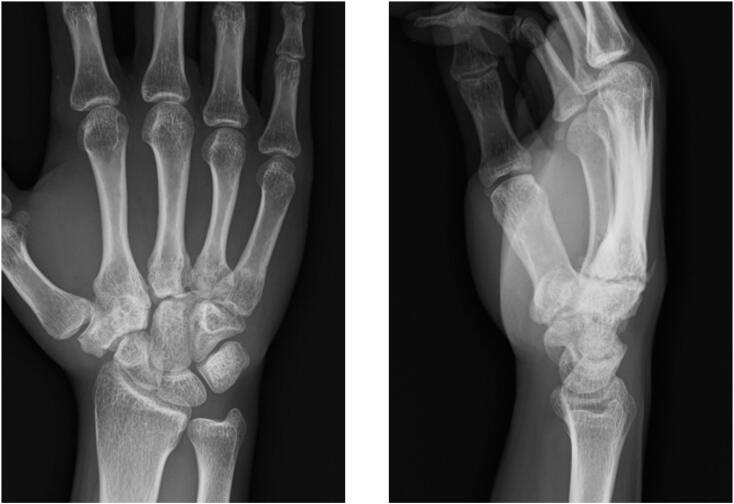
X-rays at the final follow-up, 1 year postoperatively, demonstrate a healed trapezium, and well-aligned STT and CMC joints.
